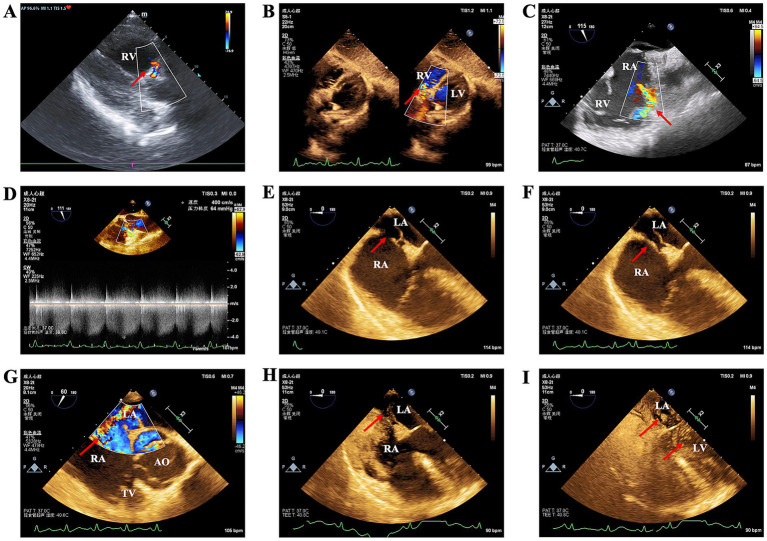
Multimodal echocardiography confirmed traumatic right coronary sinus rupture combined with atrial septal tear. (A) TTE revealed an abnormal flow jet from the right coronary sinus shunting into the right ventricle. (B) TTE revealed a similar abnormal shunt in the subxiphoid view. (C) TEE showed the rupture of the right coronary sinus into both the right atrium and right ventricle. (D) TEE exhibited a pansystolic and pandiastolic shunt on color Doppler with a velocity of 400 cm/s and a maximum pressure gradient of 64 mmHg. (E,F) TEE showed the separation of the two atrial septal layers, with a flail flap bulging and fluttering into the left atrium. (G) TEE exhibited a bidirectional shunt at the atrial level on color Doppler. (H,I) ASCE demonstrated rapid left-heart opacification after contrast agent entered the right heart, with numerous microbubbles crossing from the atrial level into the left atrium and subsequently the left ventricle. ASCE, agitated saline contrast echocardiography; TEE, transesophageal echocardiography; TTE, transthoracic echocardiography.
